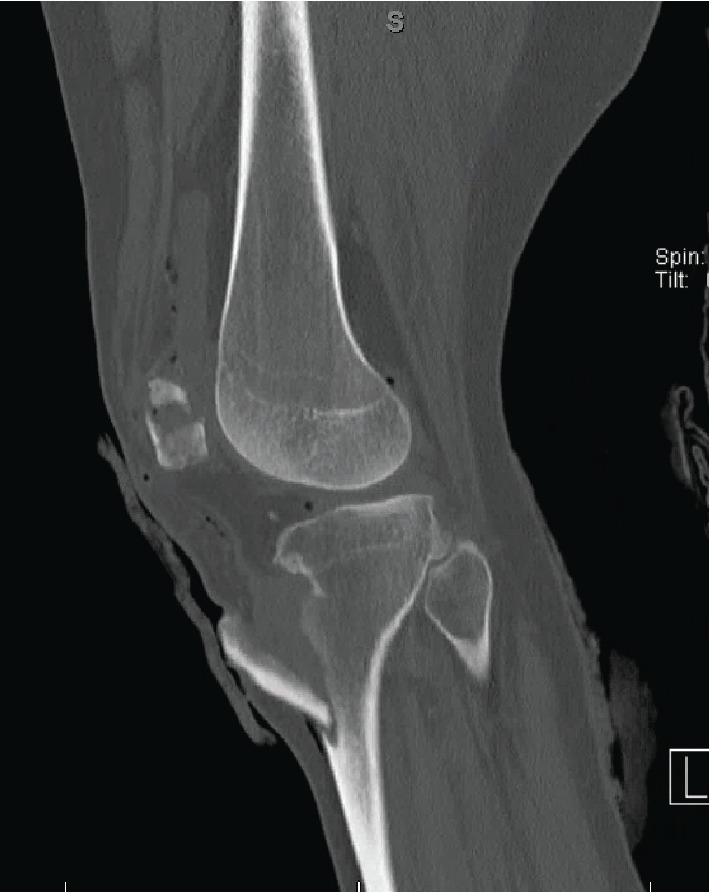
CT scan delineating the vertical, coronal plane fracture line of the extra-articular tibial tubercle fracture with overlying soft tissue injury. Also demonstrates a large bony defect in the patella, confirming the comminute nature of the fracture.
